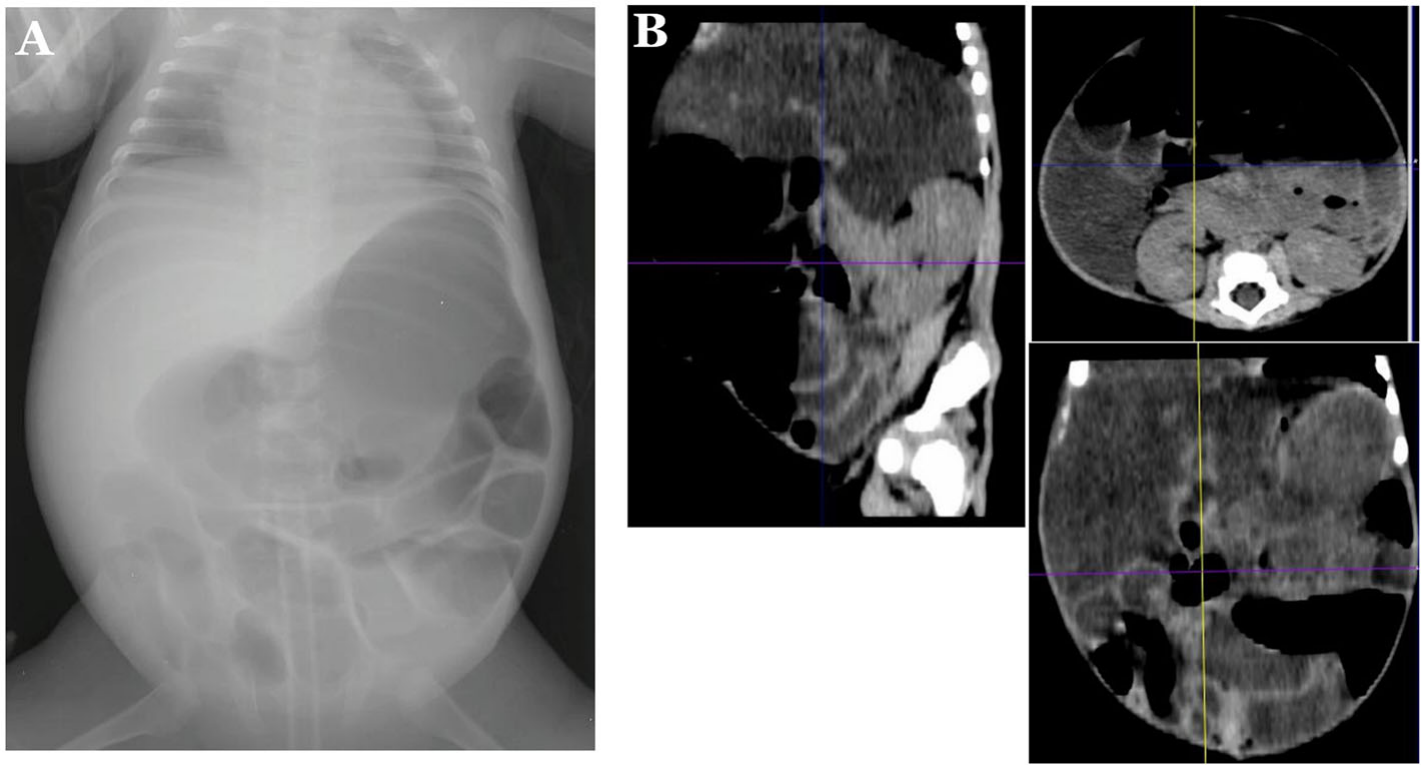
Plain abdominal x-ray (a) and CT scan (b) showed gastric and intestinal dilatation and gasless condition at the colon, suggesting a small bowel obstruction, and bowel wall thickening indicating peritonitis, without any free subdiaphragmatic air (pneumoperitoneum)
